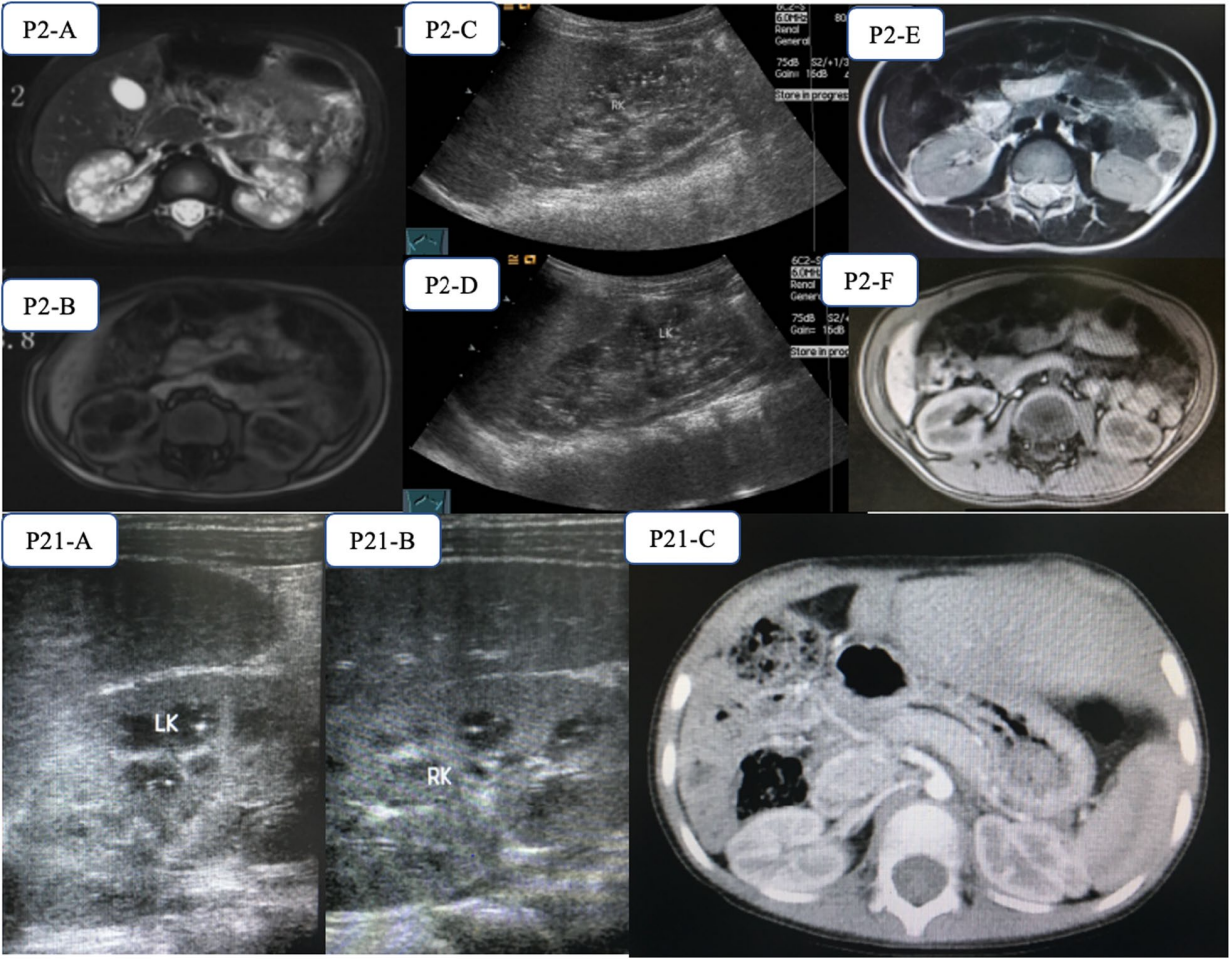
Evolution of renal lesions. In patient P2: before the commencement of chenodeoxycholic acid (CDCA) administration, diminished corticomedullary differentiation and multiple small cystic were revealed with high signal on T2WI-FS (P2-A) and low signal on T1WI-Flash (P2-B) in bilateral renal medulla by MRI. This presented as bilateral renal sponge-like degeneration with point-like deposition of calcium salts seen by renal ultrasound (P2-C and P2-2D). After CDCA treatment for a period of about 33 months (2012.2–2014.11), MRI showed disappearance of the renal lesions in this patient (P2-E and P2-F). In patient P21: calcium deposition in both kidneys was noticed by ultrasound (P21-A and P21-B) at first referral, and normal kidneys were shown by CT scan 18mo after liver transplantation (P21-C)
